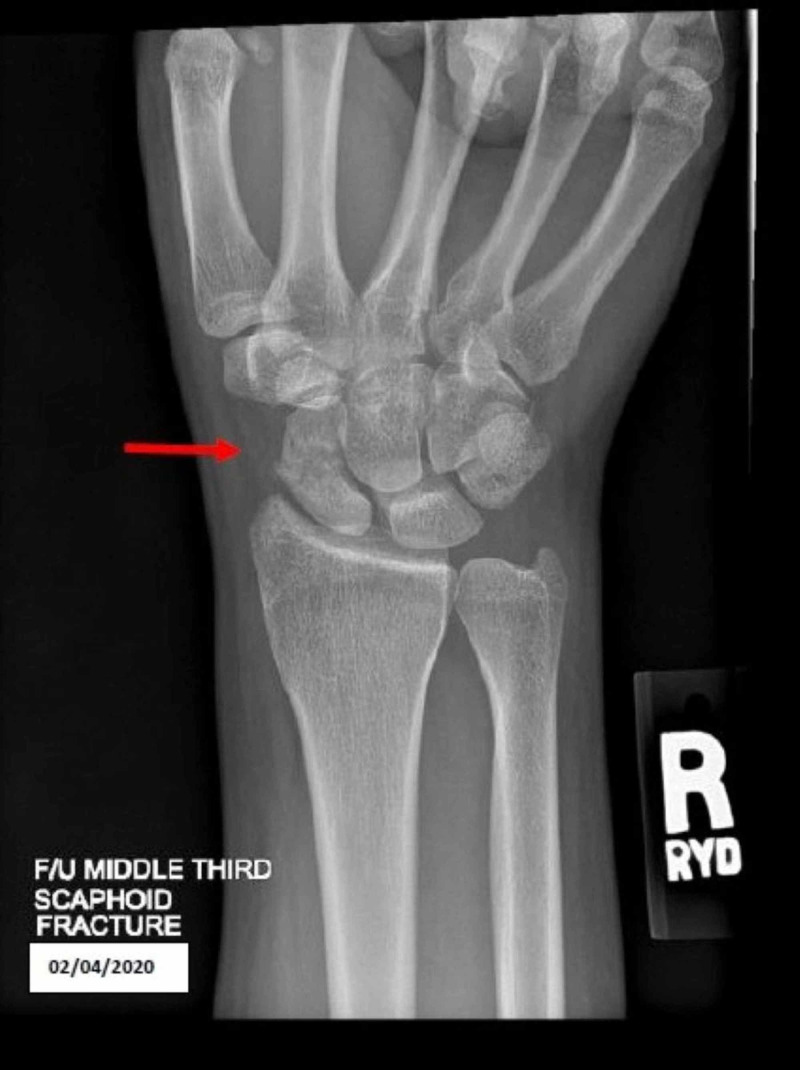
X-ray revealed stable alignment of healing fracture of the scaphoid with an interval increase in osseous bridging compared to prior (Red arrow)
